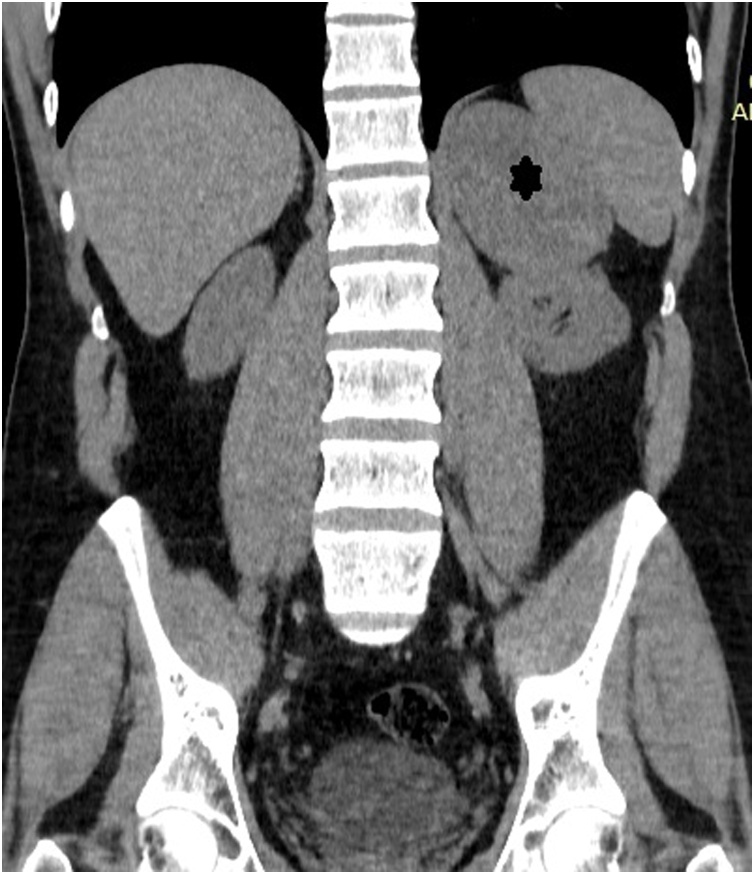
CT abdomen/pelvis revealing a 8 × 5 cm left adrenal mass(Asterick).
